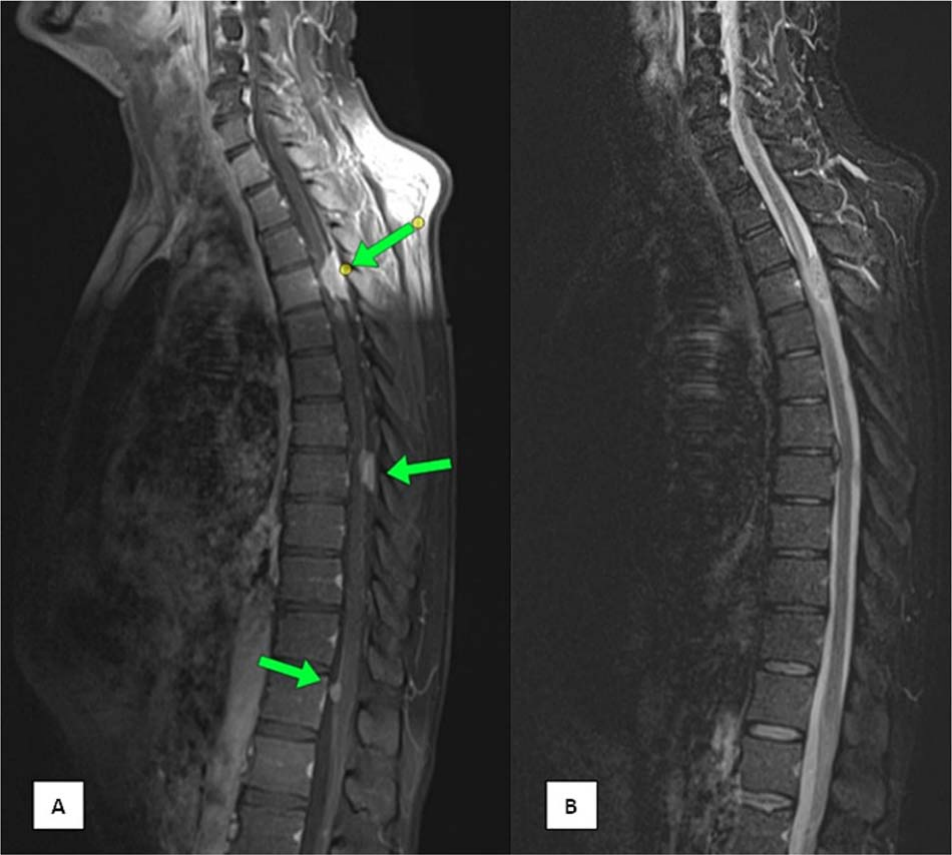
MRI of the spine (A) sagittal T1-weighted fat-saturated post-contrast image and (B) sagittal Short Tau Inversion Recovery (STIR) sequence demonstrating multiple intra-spinal, extra-medullary meningeal-based enhancing lesions. The largest lesion measures 22 × 11 mm at the D4 level.
